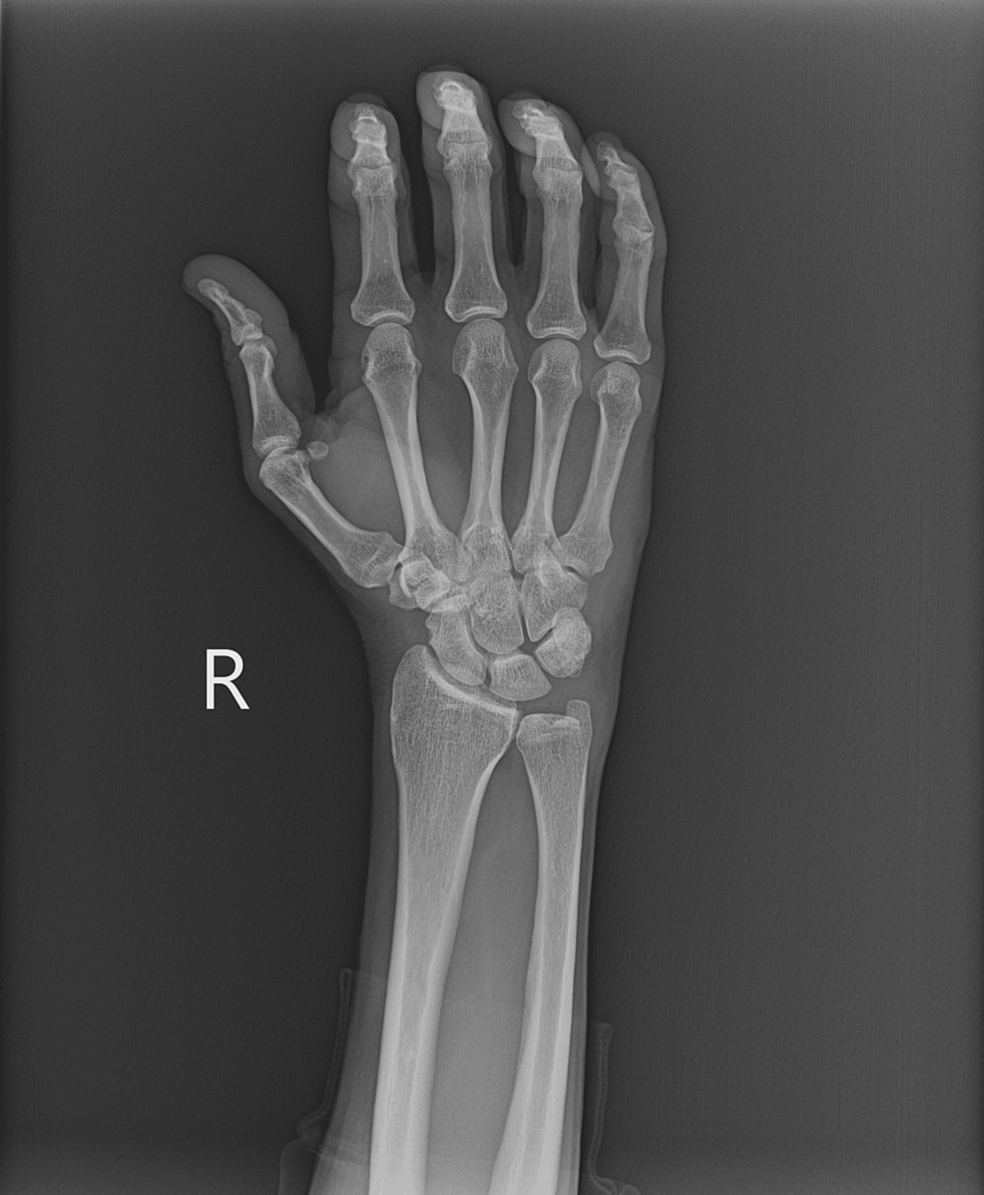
Posteroanterior View Right Wrist X-ray
